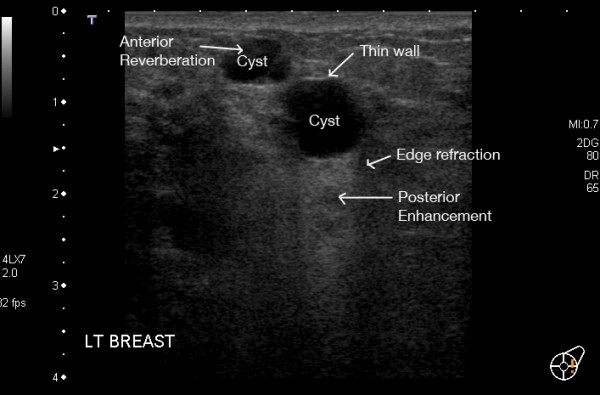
Ultrasound image of multiple cysts in the left breast of a lactating woman. The cysts display typical criteria such as thin walls, posterior enhancement, edge refraction and anterior reverberation.
